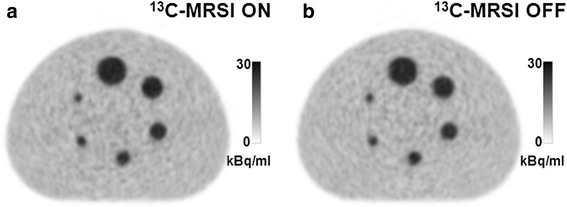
Examples of PET during ^13^C-MRSI ON and OFF. PET with a simultaneous ^13^C-MRSI (scan number 1) and b without any MRI performed (scan number 2)
